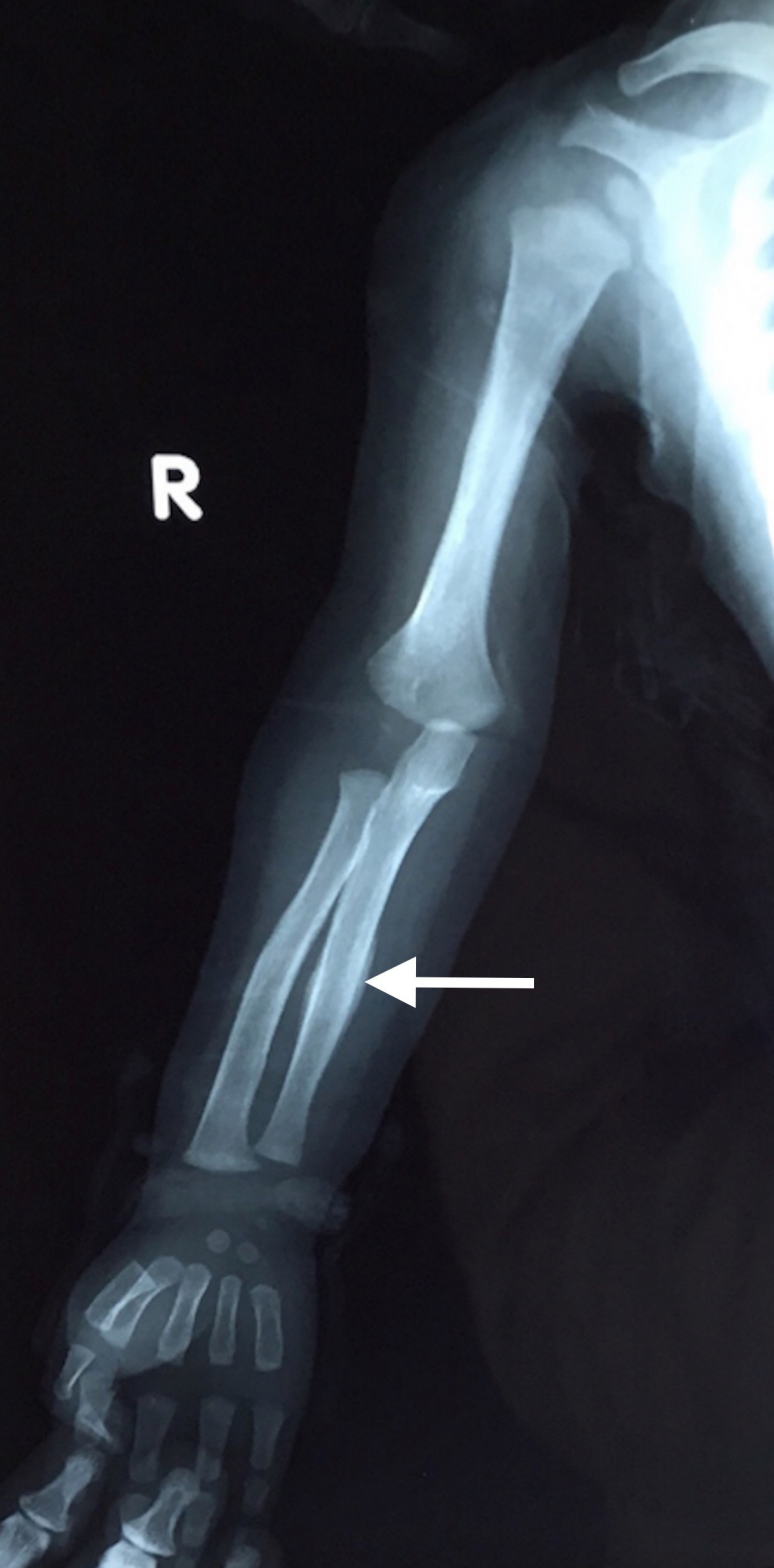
Anteroposterior (AP) view of right upper limb Radiograph shows similar laid down lamellated periosteal reaction involving lateral and medial cortex of ulna (arrow).
